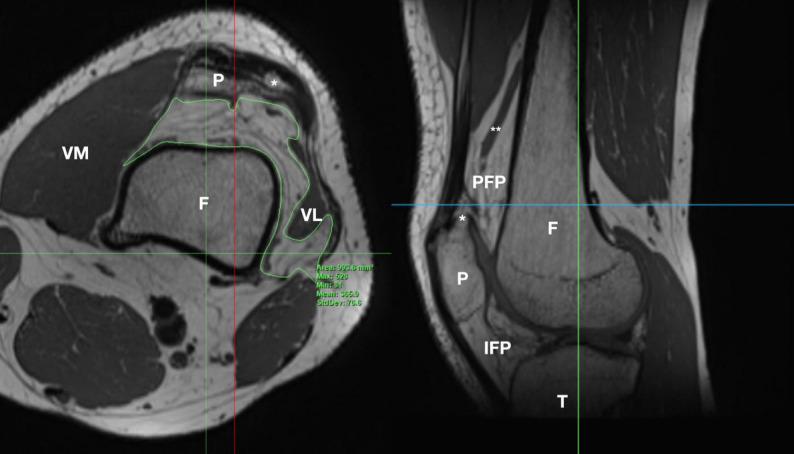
Suprapatellar axial PFP area (SAPA) measurement. Left: Axial MRI at the level of the suprapatellar recess demonstrating the outlined PFP area (SAPA, green). The PFP lies adjacent to the medial and lateral vastus muscles (VM and VL), the femur (F) and the patella (P). The SFP (*) is separated from the PFP via the suprapatellar recess. Right: median sagittal view of the knee. The blue line indicates the level of the corresponding axial view. The PFP lies posterior to the patella (P) and the SFP (*) and anterior to the femur (F). The articularis genus muscle () extends into the PFP and its strands were excluded from SAPA area measurement. The PFP does not communicate directly with the IFP or the tibia (T)
